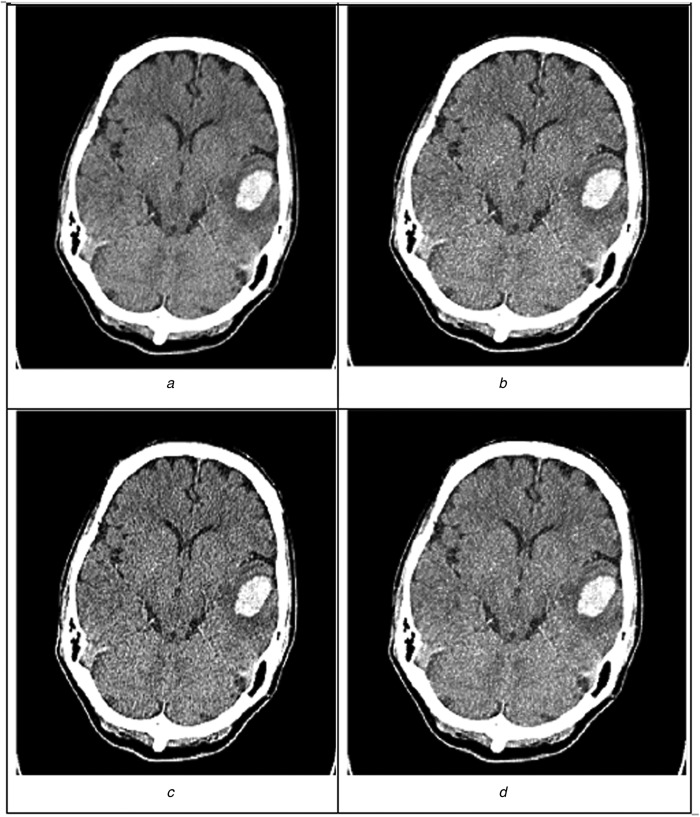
Image showing the image advancement techniques implemented on CT image *a* Original grey-scale image *b* Wiener filter with modified UM *c* Non-local means with modified UM *d* Image denoised with a wavelet and enhanced with modified UM
